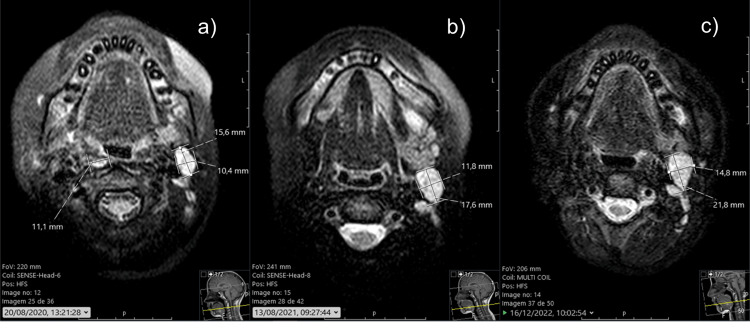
(a) MRI in August 2020; (b) MRI in August 2021; and (c) MRI in December 2022.
